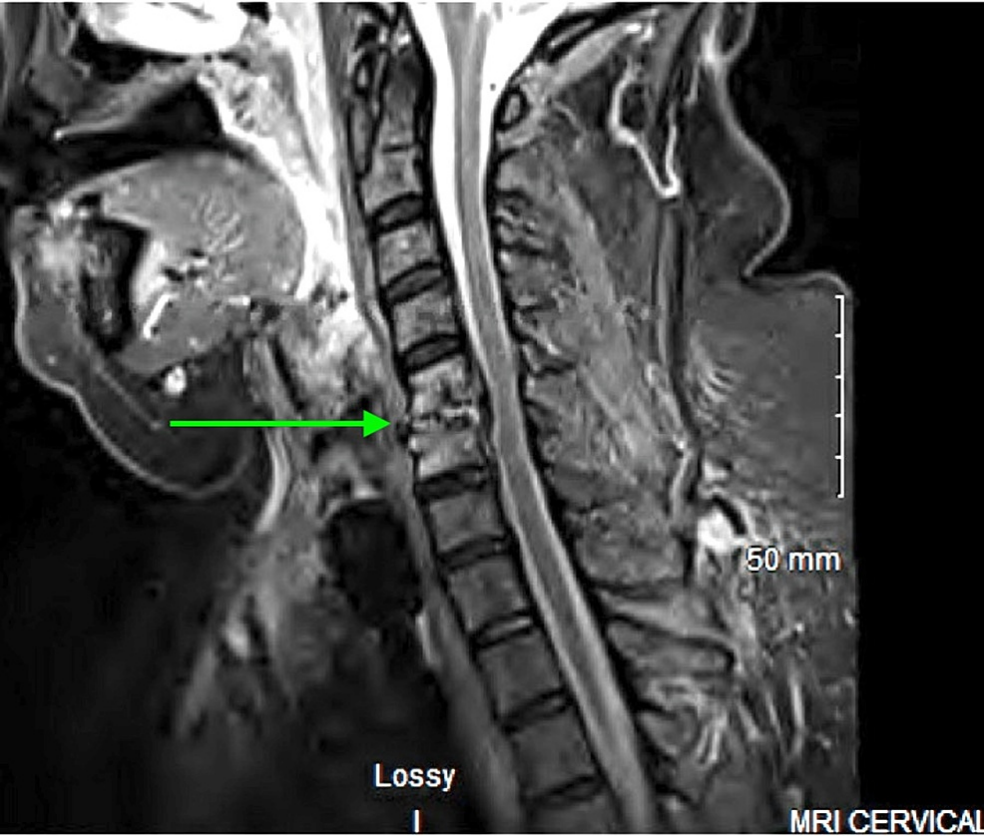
STIR-sequence cervical spine MRI demonstrating C5-C6 osteomyelitis STIR: short tau inversion recovery; MRI: magnetic resonance imaging
